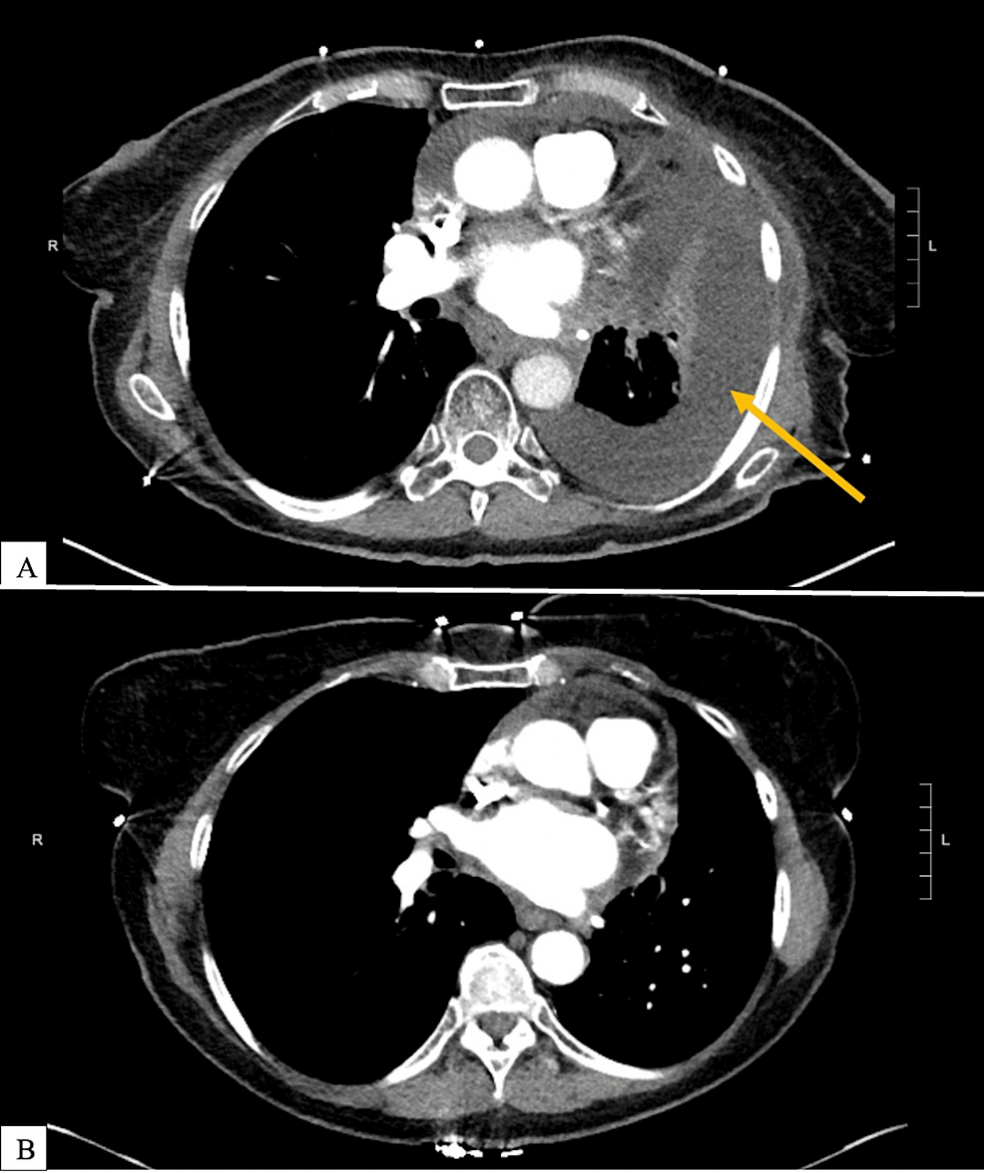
CT pulmonary angiogram at the time of hospital presentation (a) revealing a large loculated left pleural effusion (arrow), with near complete collapse of the left lower lobe. A CT chest from approximately the same level from seven months prior (b) showing only minimal pericardial effusion and absence of pleural effusion or atelectasis.
